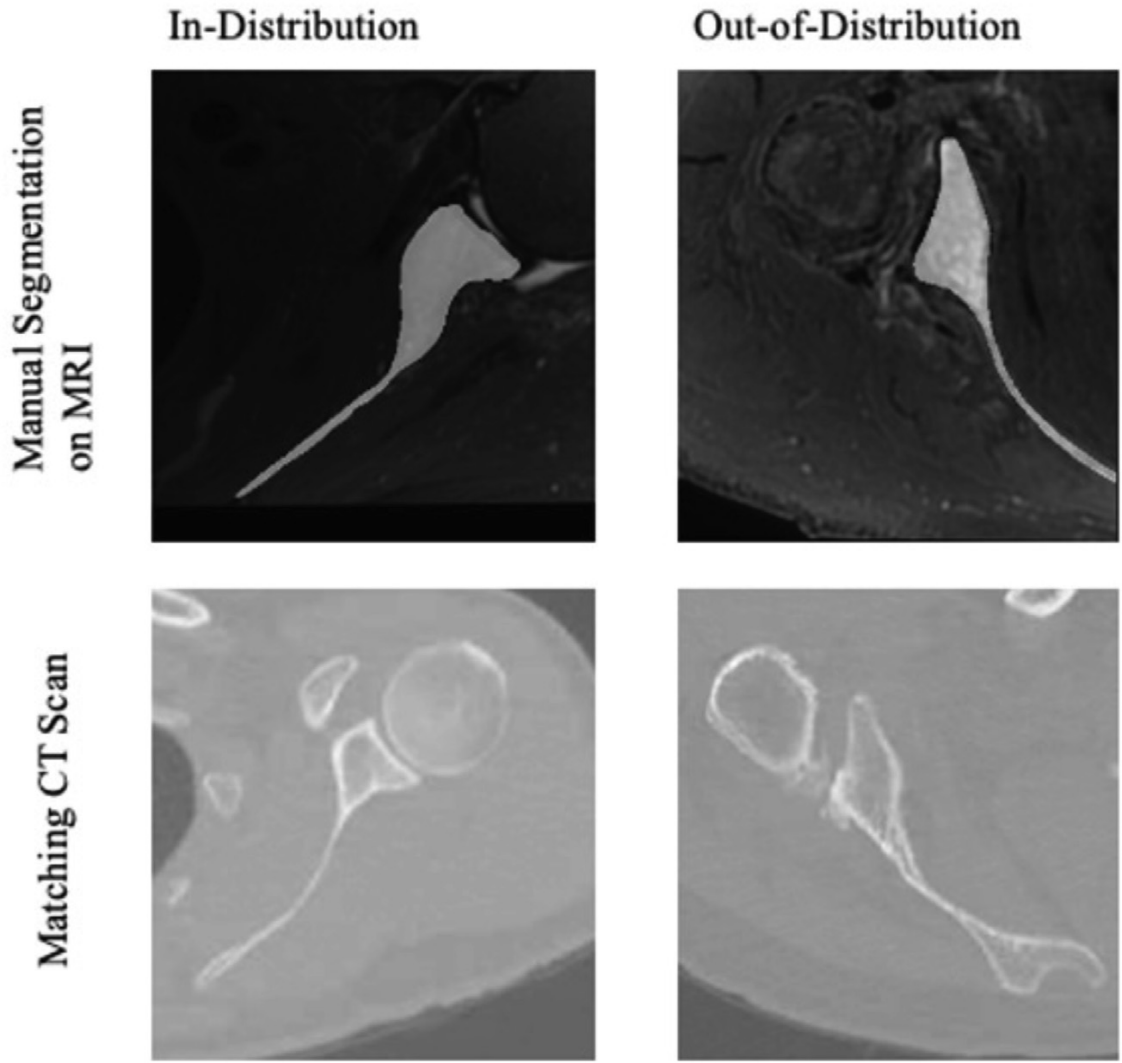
Manual segmentation overlayed on MRI and corresponding CT scans for in-distribution and out-of-distribution patients in our test set. *MRI*, magnetic resonance imaging; *CT*, computed tomography.
